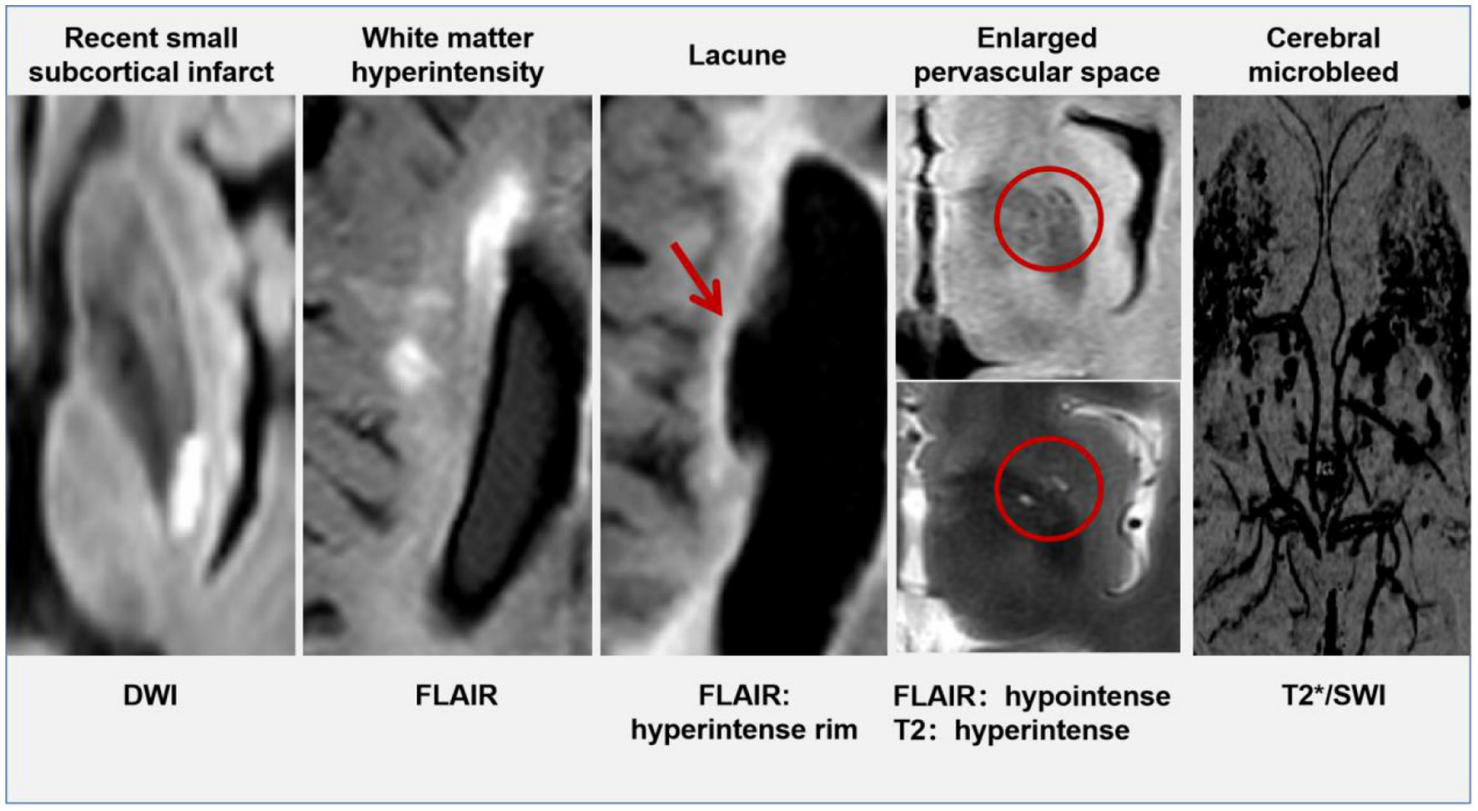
The typical manifestation of cerebral small vessel disease (CSVD) on MRI.
